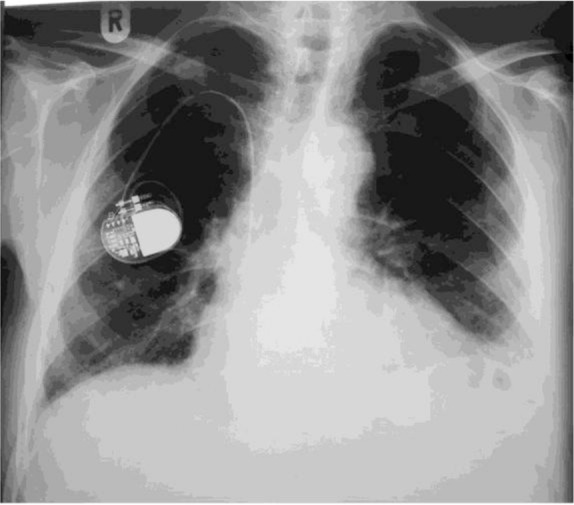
Repeat chest X-ray after 4 days of treatment for heart failure, showing clearance of lung field
